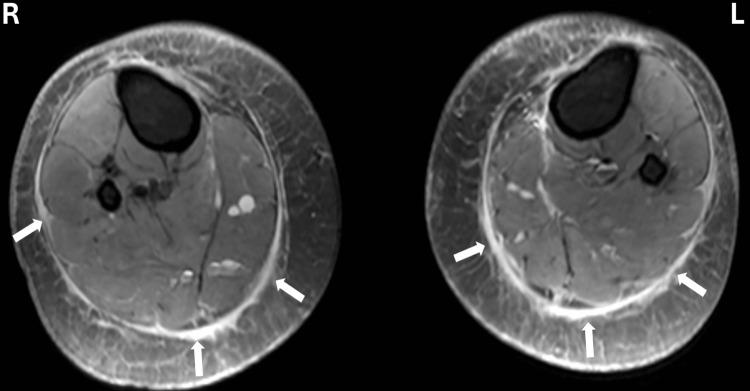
Axial T2 MRI of the lower limbs showing fascial thickening (arrows)
